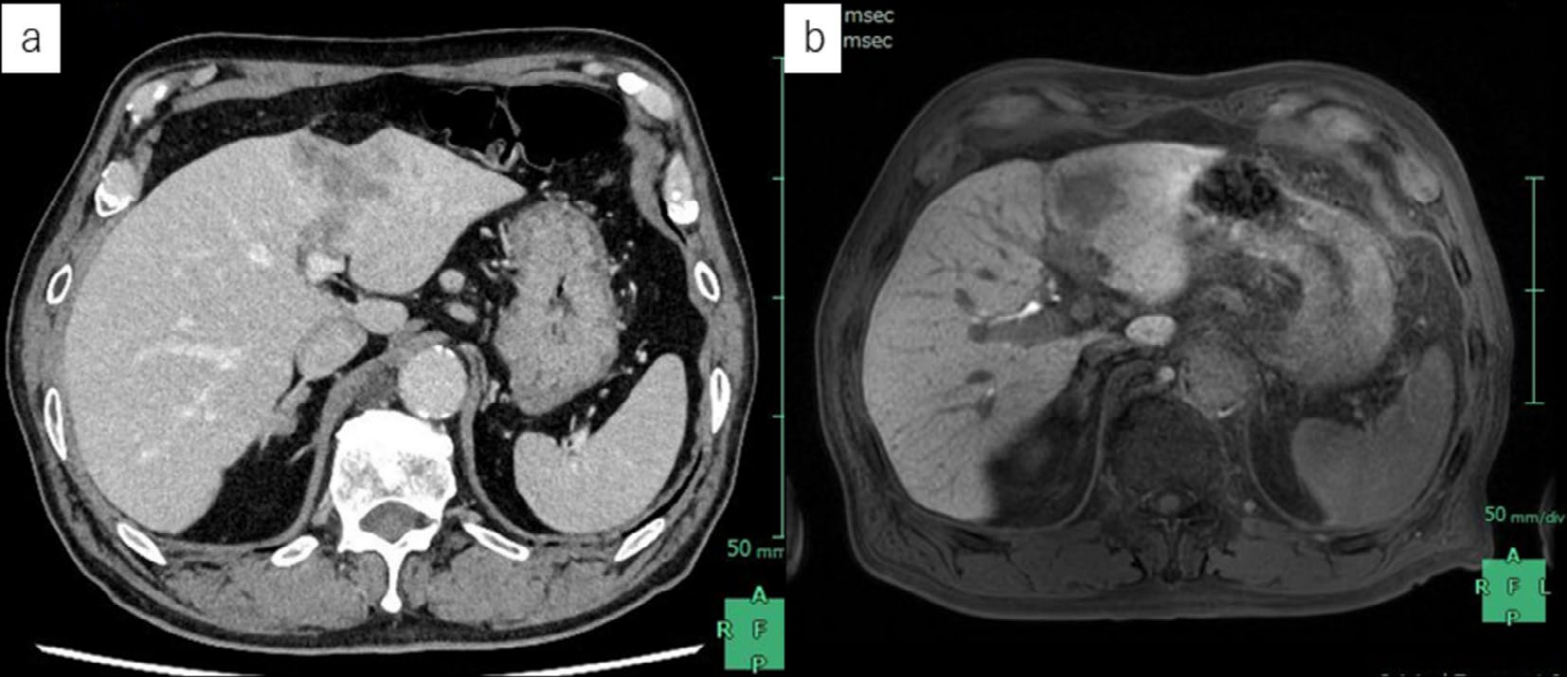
Preoperative imaging shows a 46‐mm tumor in liver Segment 3. (a) Contrast‐enhanced computed tomography. (b) Contrast‐enhanced magnetic resonance imaging.
